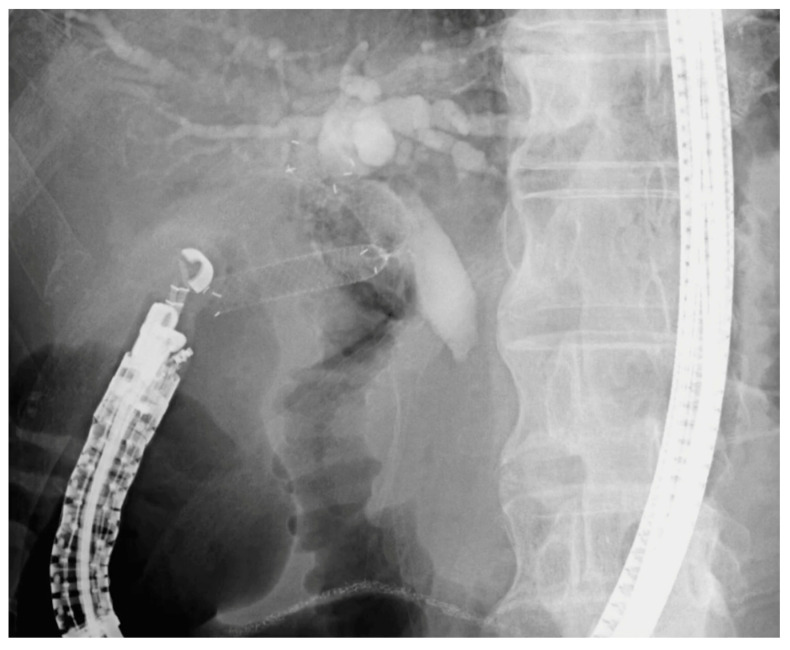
Fluoroscopic image of EUS-guided choledochoduodenostomy (EUS-CDS). A fully covered self-expandable metal stent is deployed from the duodenal bulb into the common bile duct under endosonographic and fluoroscopic guidance for biliary drainage in a patient with malignant distal biliary obstruction.
